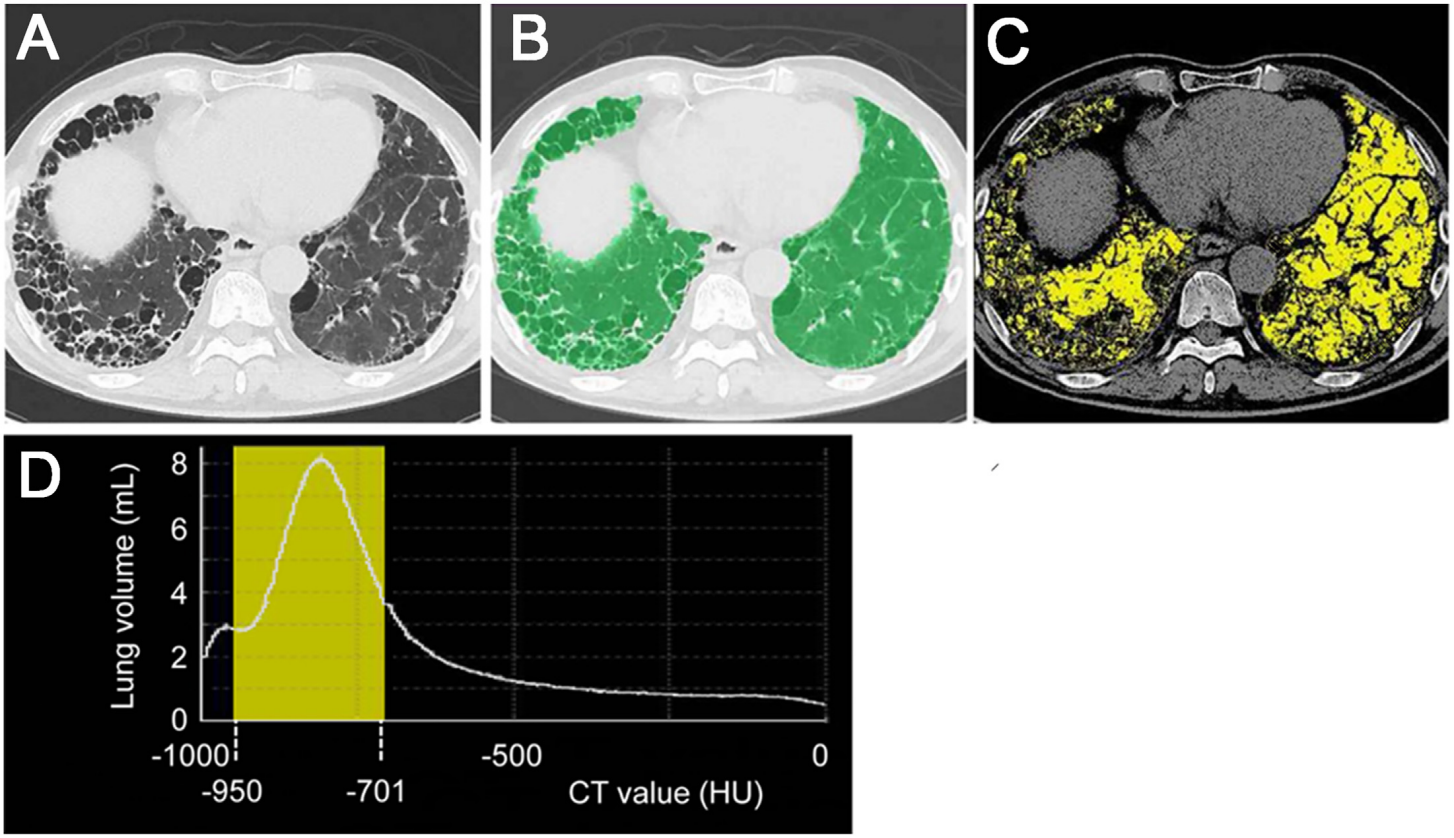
Volumetric analysis of normally attenuated lung. Chest computed tomography (CT) image of a 79-year-old man with IPF showing UIP pattern. This CT slice shows peripheral honeycomb lung predominantly on the right side (A). The CT image showing extracted whole lungs (in green) (B). The volume of the whole lungs (WL) is 2,438 milliliter (mL). The CT image showing normally attenuated lung, as defined between −950 Hounsfield units (HU) and −701 HU (in yellow) (C). The volume of normally attenuated lung (NL) is 1,346 mL. Although some parts of honeycombing are marked as normally attenuated lung (in yellow) by the workstation, most areas of normally attenuated lung are considered to be identical to normal lung tissue. The yellow area under the histogram curve represents the NL (D). The percentage of NL (NL%) is 55.2%. Forced vital capacity and diffusing capacity of the lungs for carbon monoxide of this patient are 1.59 L (52.1%, %predicted) and 6.6 mL/min/mmHg (59.2%, %predicted), respectively. His partial pressure of arterial oxygen is 65.9 Torr, and the lowest percutaneous oxygen saturation is 84% during the 6-min walk test. The patient was classified in Japanese stage IV and GAP stage II.
